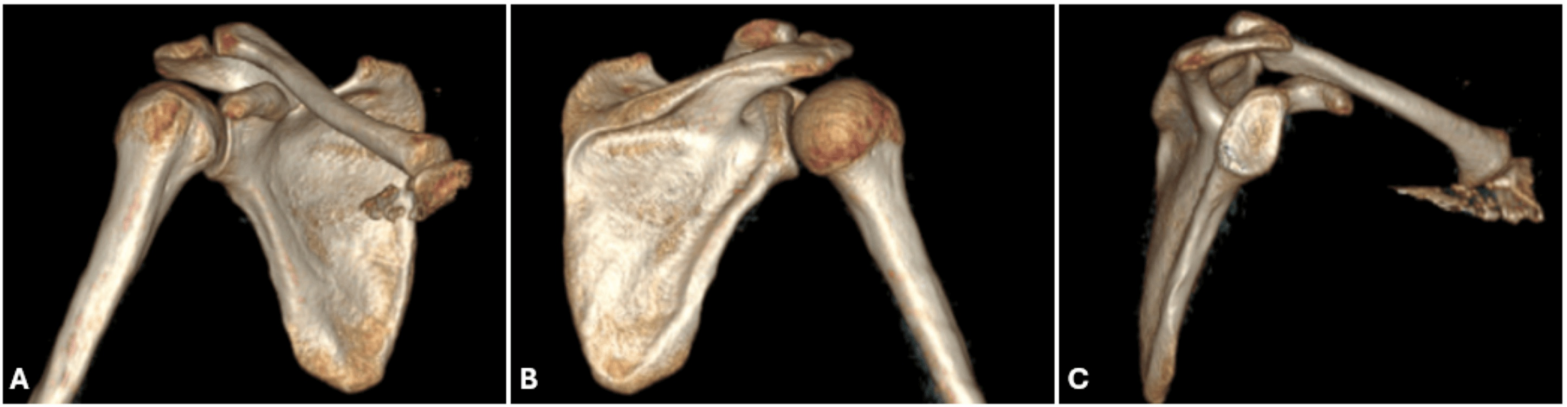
Three-dimensional computed tomography reconstructions of the right shoulder demonstrate an intact glenohumeral joint in the anterior (A) and posterior (B) views and an intact glenoid after the humerus was distracted (C).
